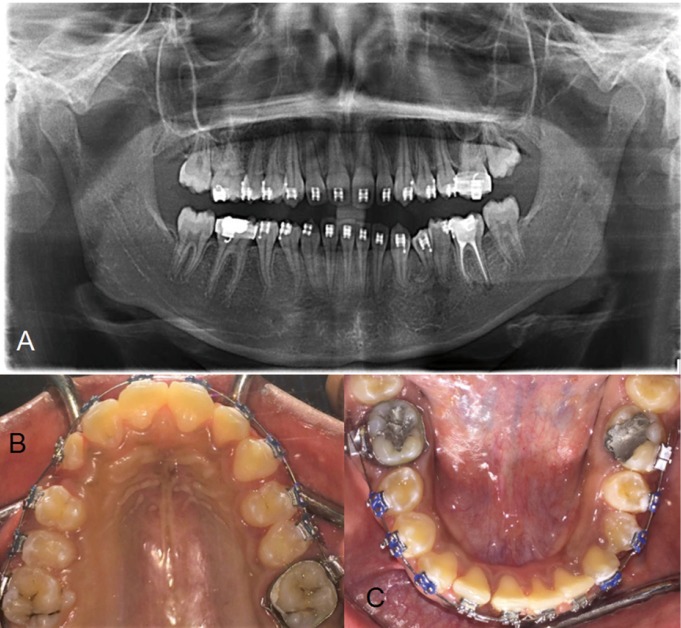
A) Panoramic radiograph after alignment of teeth and healing of the cystic cavity. B) Eruption of teeth in the upper arch which still need slight alignment. C) Uneventful eruption, and alignment of the impacted teeth in the lower arch.
